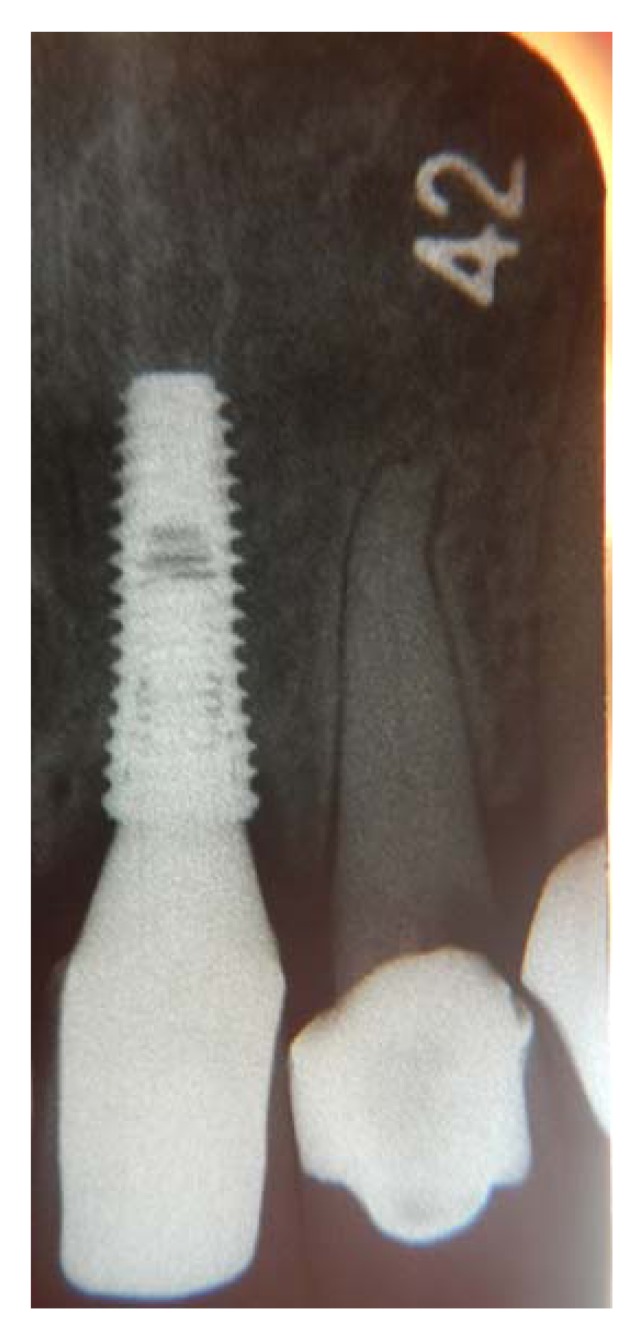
Periapical radiograph at the time the crown was placed shows a good crestal bone level.
